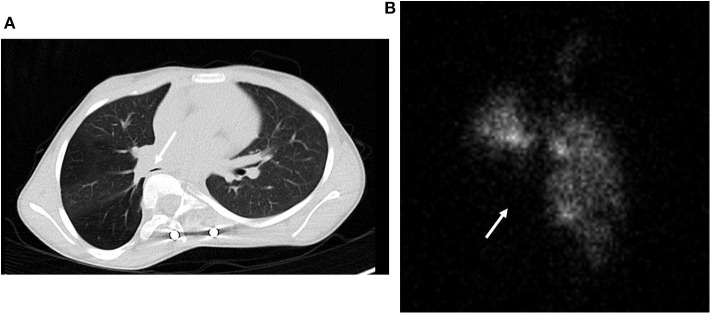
(A) CT scan of lobar bronchial compression by the spine due to scoliosis and vertebral intrusion into the thorax. (B) Lung ventilation scan correlated with the CT scan in (A) demonstrating loss of ventilation in the right middle and lower lobes.
